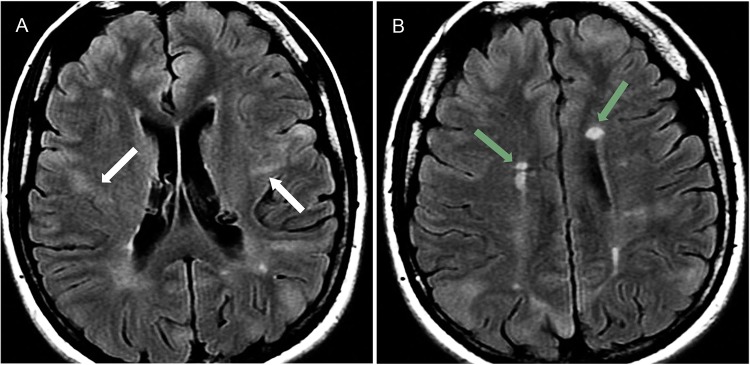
Tuberous sclerosis in a 21-year-old female with drug-resistant partial epilepsy. A) Axial FLAIR images show hyperintense cortical tubers with a band of abnormal signal intensity from the cortex to the lateral ventricle (white arrows) and B) multiple subependymal hamartome (green arrows) with a hyperintense signal.
